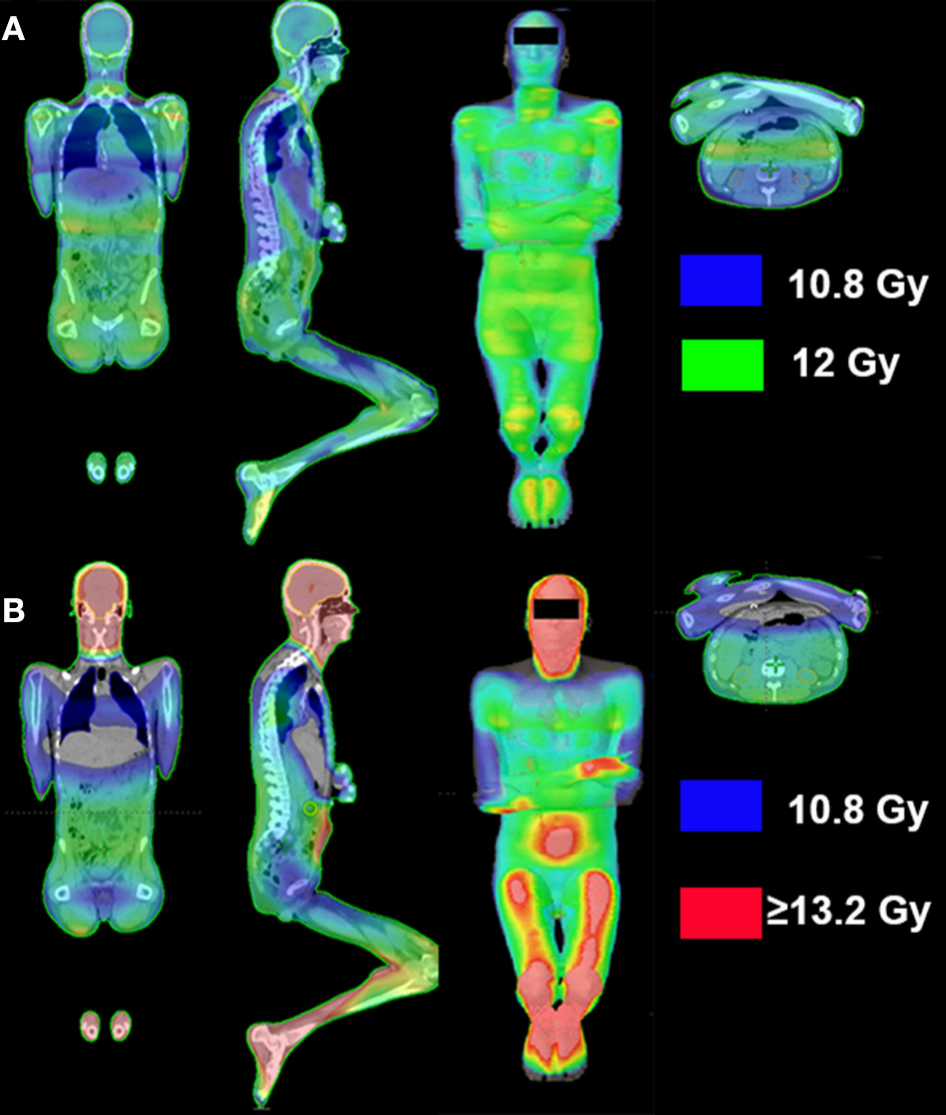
Conventional vs. SSD IMRT-planned total body irradiation dose distribution. (A) Computed tomography (CT)-planned, image-guided intensity-modulated radiotherapy (IMRT) dose distribution with lateral-beam setup at source-surface distance (SSD) (123); dose reductions were planned over lungs, and kidneys. (B) CT-planned two-dimensional conventional total body irradiation dose distribution with a lateral-beam setup, with lung dose reduction using lung blocks; the isowash-depicted dose range in the images represents 90% (10.8 Gy; blue) to ≥110% (≥13.2 Gy; red) of the prescribed dose.
